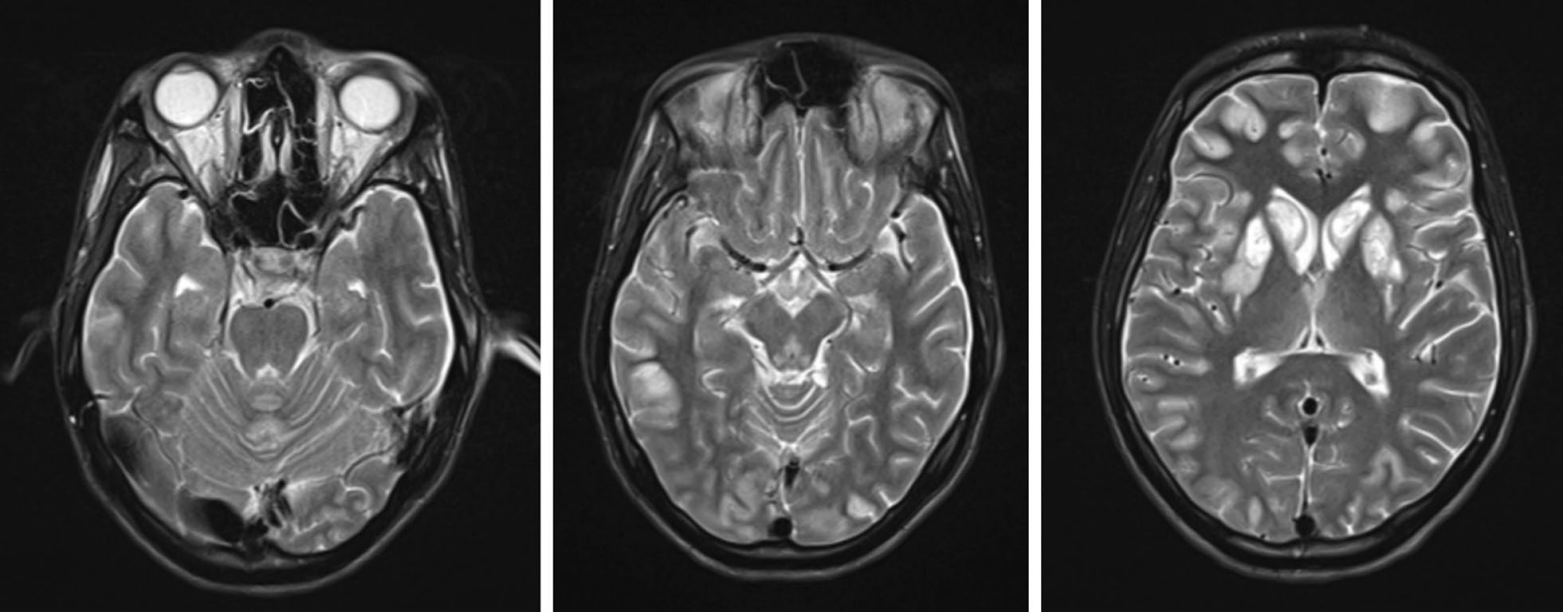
MRI of the brain. Narrowing of the subarachnoid space. A pathological increase in the intensity of the signal by T2/TIRM and DWI from the cortex of the brain substance is determined, the change in the signal is symmetrical from the basal nuclei with their hemorrhagic transformation. Symmetrically increased T2/TIRM signal from the medial thalamus, periaqueductal gray matter
